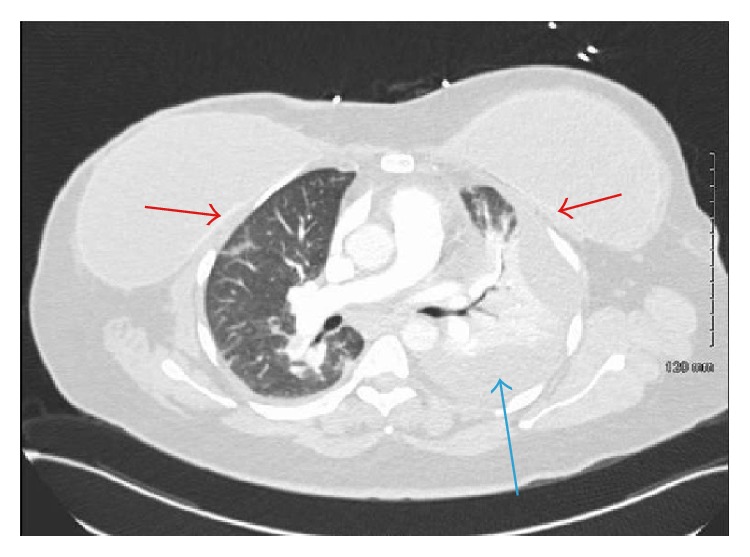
Contrast CT of the thorax showing moderate size left sided pleural effusion (blue arrow) and bilateral silicone implants with intact capsule (red arrows).
